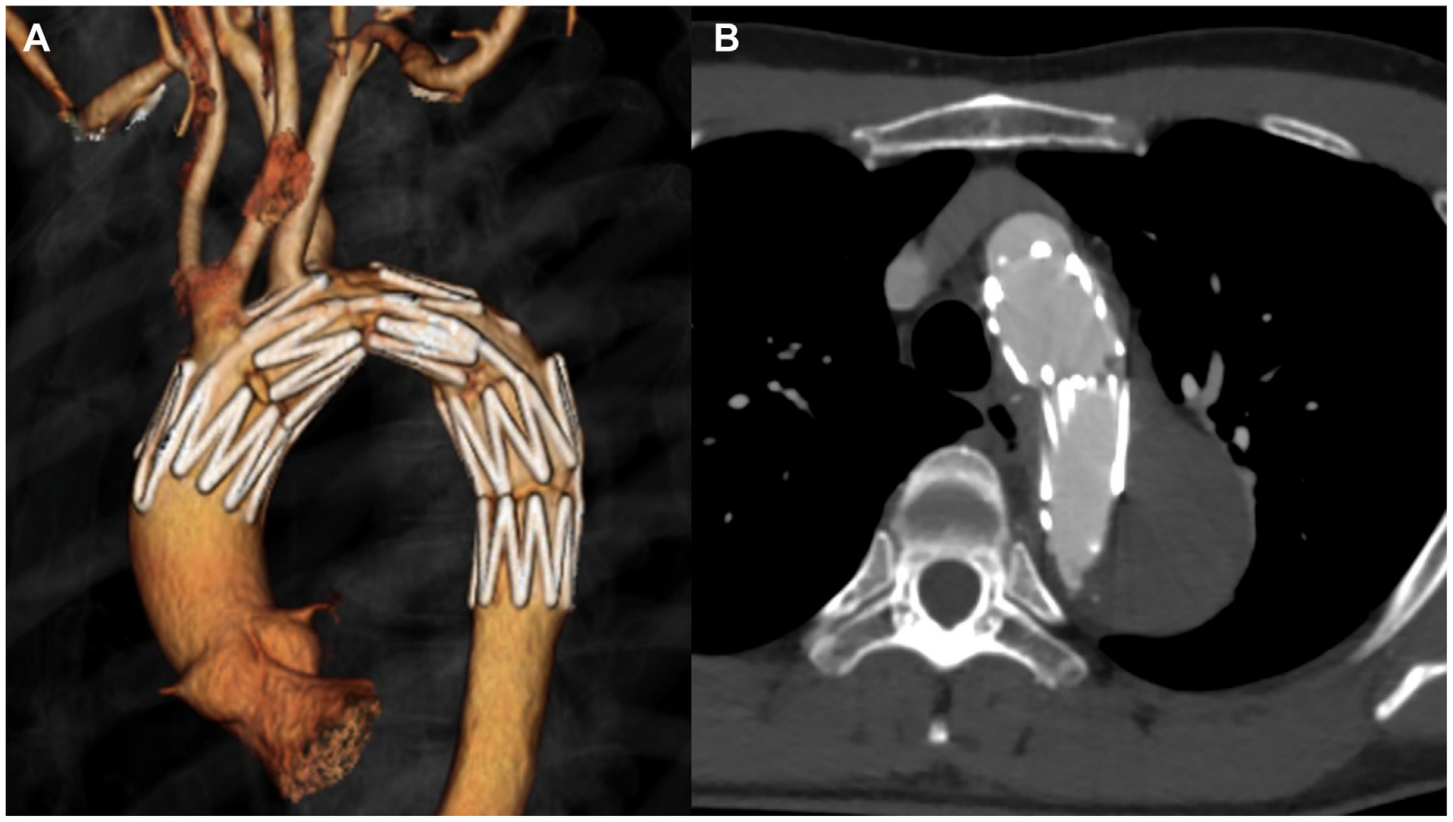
Postoperative contrast-enhanced computed tomography (CT) images. (A and B) Postoperative contrast-enhanced CT revealed entry closure and patency of the four neck vessels.
